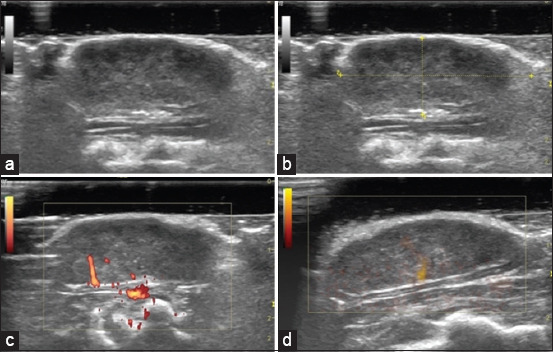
(a and b) Monitoring of mammary tumors by ultrasonography, using B-mode, (c and d) Power Doppler and B Flow. (b) The tumor length and depth can be measured using the using electronic cursors integrated into the ultrasound machine and set at the borders of the tumor [Source: Figure prepared by the authors].
